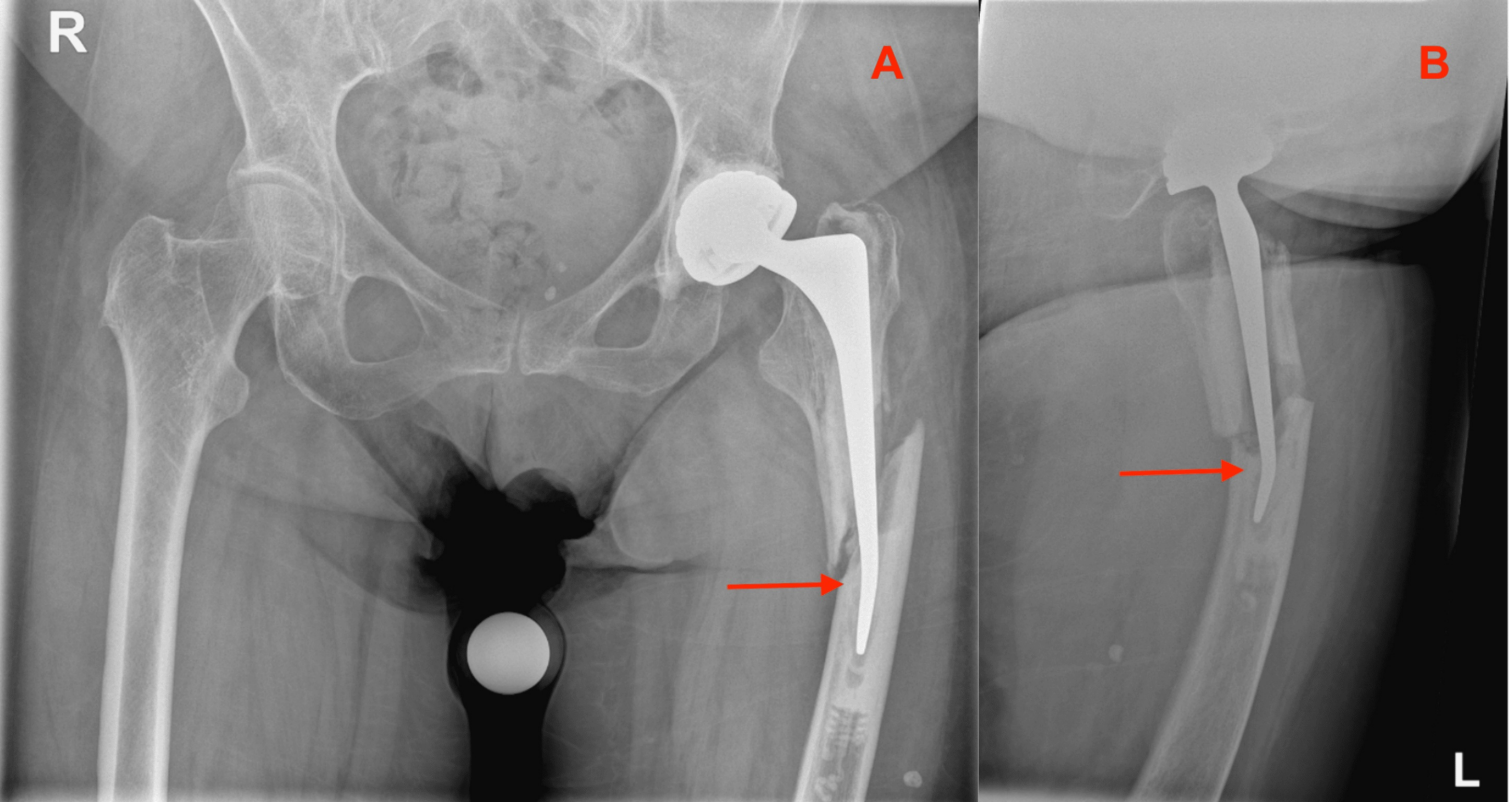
Vancouver B2 periprosthetic fracture with CPT stem bending (arrows) Radiographs of the pelvis and left hip performed at the emergency department where the patient was seen with complaints of left-sided hip pain. 3A: Anterior Posterior view of the Pelvis; 3B: Lateral view of the Left Hip CPT: Collarless, Polished, Double-Taper
